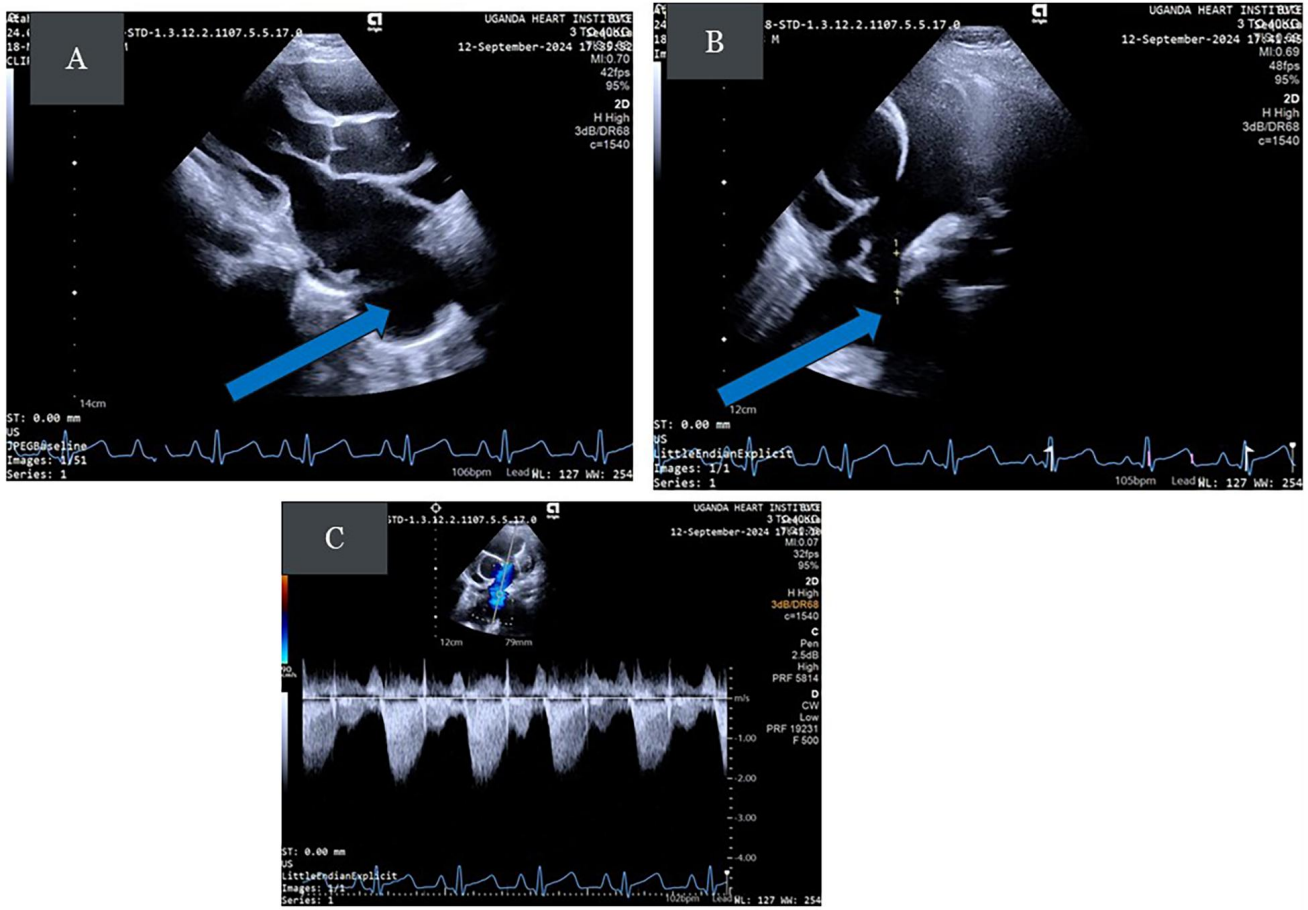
(A) Parasternal long-axis (PLAX) view demonstrating the dilated LA and a large aneurysm posterior to the left atrium (bold arrow). (B) Modified parasternal short-axis view showing a dilated RPA and a fistula connecting the RPA (small arrows) to the aneurysm (bold arrow). The pulmonary artery may dilate due to either increased flow or increased pulmonary artery pressure. (C) Doppler signal tracing showing the typical systolic, diastolic wave forms with a wave reversal.
